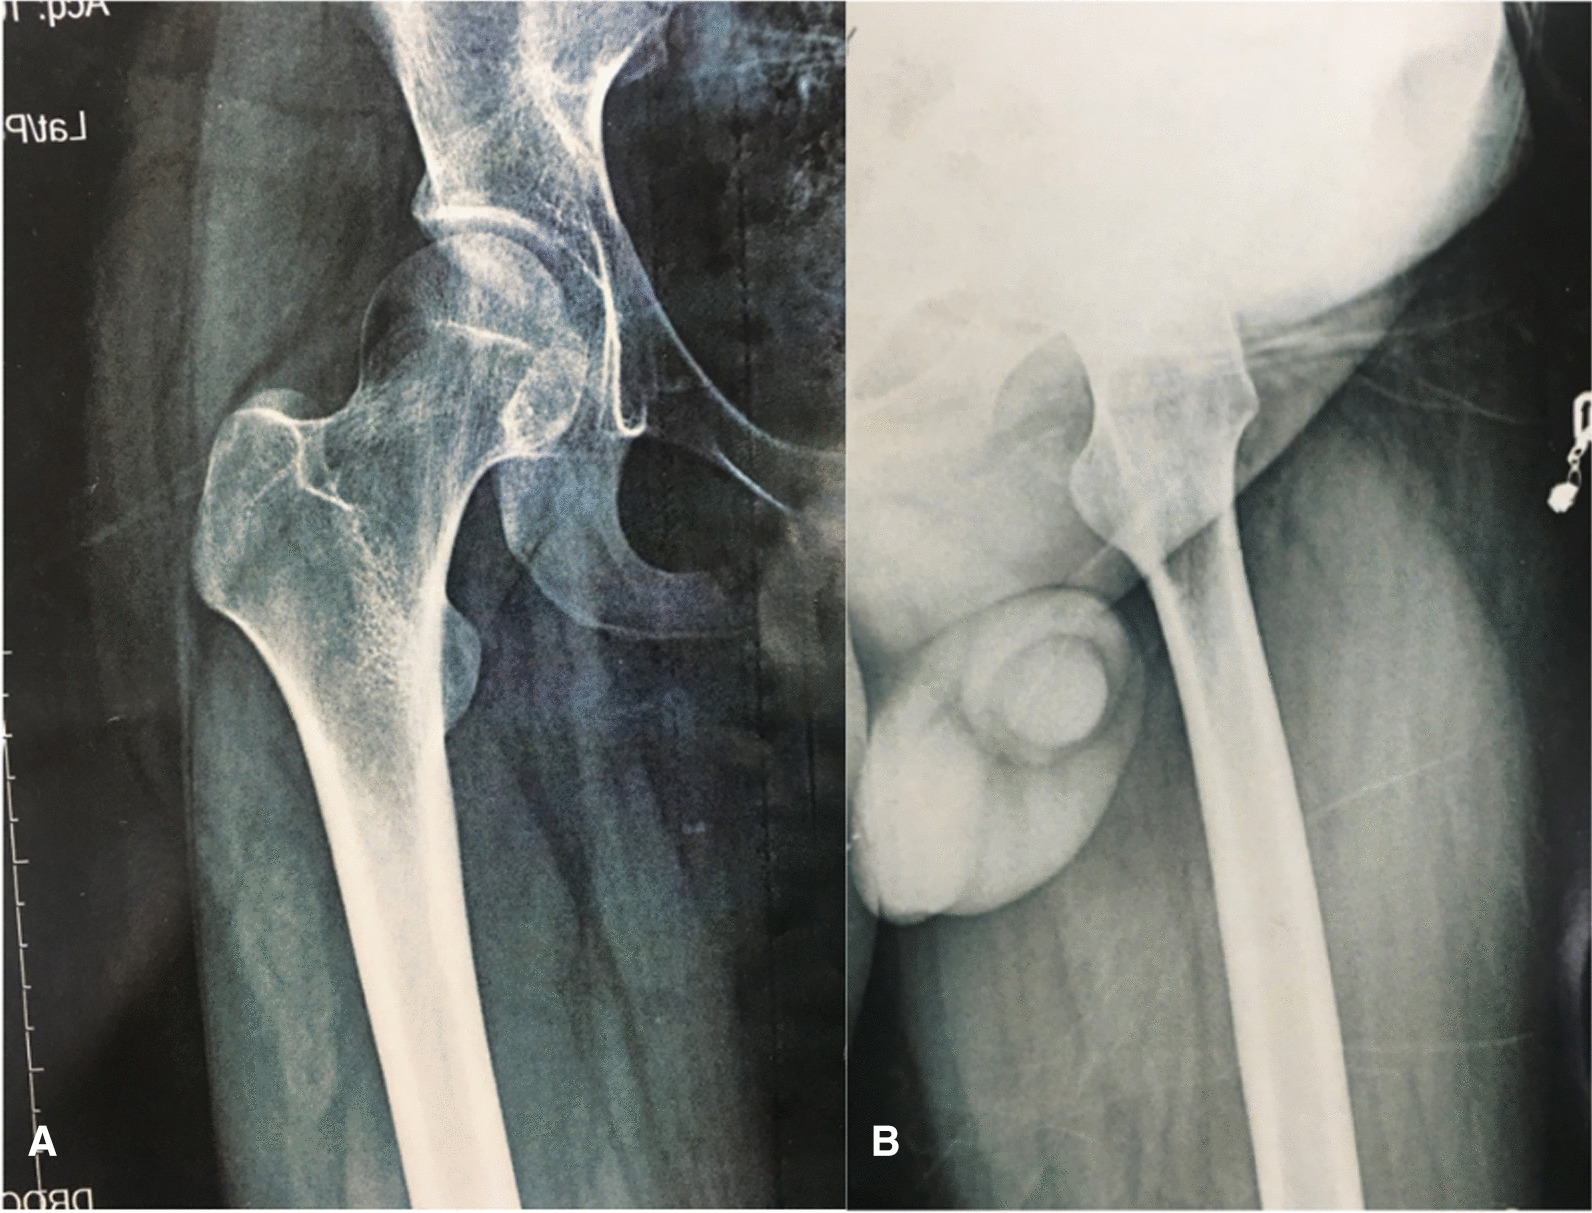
Radiographs of the right hip at initial presentation, including anteroposterior (A) and lateral (B)
